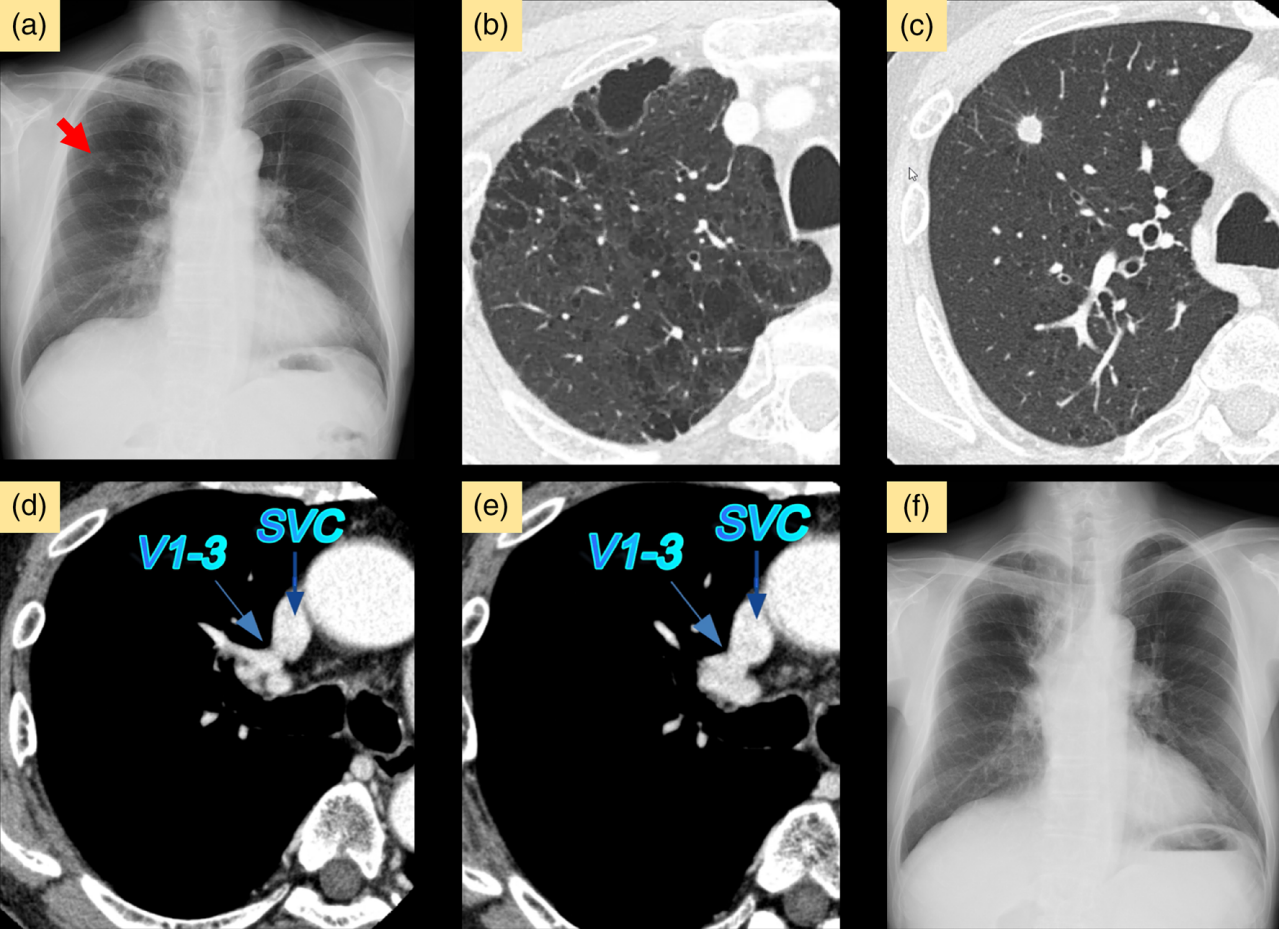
(a) Chest x‐ray revealed a nodule in the right upper lung field (red arrow) without cardiac enlargement. (b) Contrast‐enhanced computed tomography revealed severe emphysematous lung changes with diffuse low attenuation areas in the lung fields. (c) A pure solid tumor with 11 mm diameter in the S3 of the right upper lobe. (d, e) V1–3 refluxed through the right upper lobe and returned directly into the superior vena cava. (f) After 4 months following surgery, a chest x‐ray revealed that the remaining right upper lobe was well‐inflated, and no cardiac enlargement was observed. SVC, superior vena cava.
